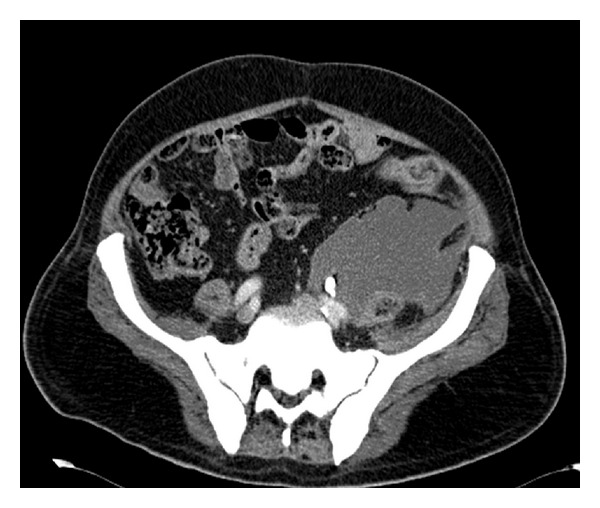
Abdomen CT scan: left urinoma, left ureteral stone, and left double J stent.
